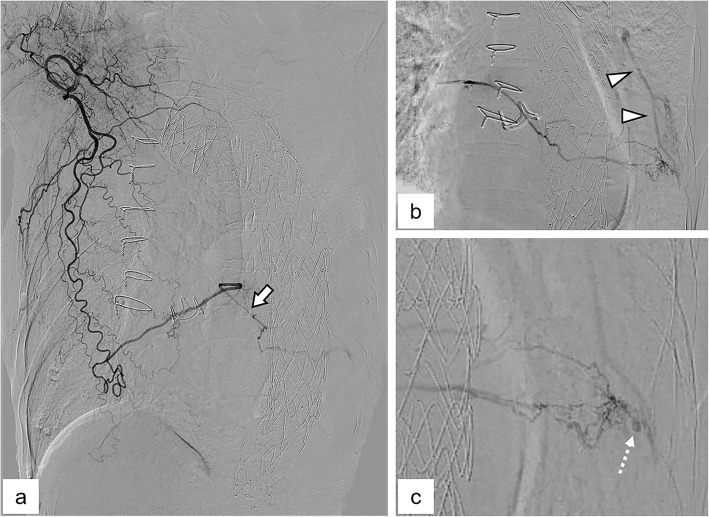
Angiographic findings. A Selective digital subtraction angiography (DSA) of the right subclavian artery. Consistent with contrast-enhanced computed tomography image, the continuity from the right subscapular artery to the left pulmonary ligament artery (PLA) (arrow) is shown. B and C Selective DSA of the left PLA. Note that the abnormal proliferation of small vessels is visible at the peripheral side of the left PLA. The arrowheads in b indicate the reversal blood flow of pulmonary artery, suggesting the presence of a systemic-pulmonary artery shunt. The dashed arrow in c indicates the pseudoaneurysm
